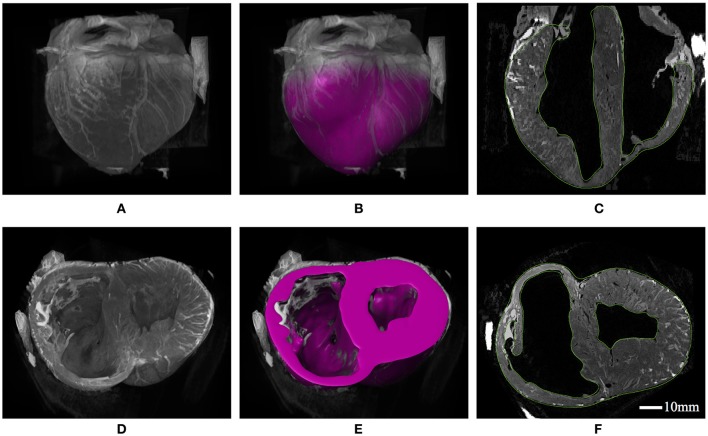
(A) 3D constructed visualizations of the full ventricular structure from MRI data. (B) Geometric segmentation of the full ventricular structure superimposed over the same background MRI data as (A). (C) Long-axis cut plane of the MRI data superimposed with contours (green lines) for the full ventricular segmentation. (D) 3D constructed visualizations of the truncated ventricular structure from MRI data, revealing the endocardial ventricular structure. (E) Geometric segmentation of truncated ventricular structure superimposed over the same background MRI data in (D). (F) Short-axis cut plane of the MRI data superimposed with contours (green lines) for the ventricular segmentation.
